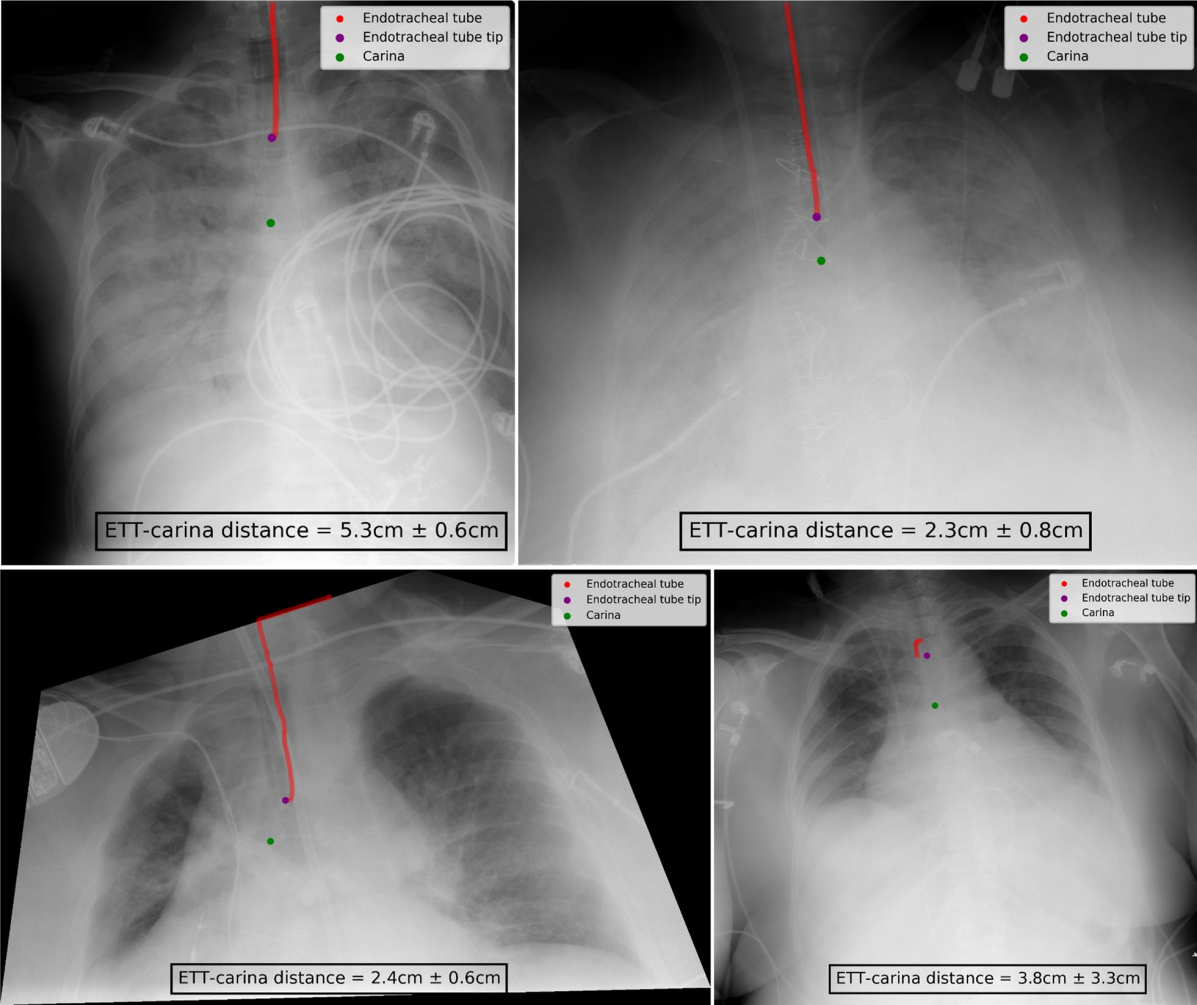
Example outputs of the CarinaNet model. Top-left and top-right chest radiograph were, respectively, classified as having *acceptable quality* and a *hardly visible carina*. The endotracheal tube tip and carina were accurately detected and the model uncertainty was inferior to 1 cm, indicating that the model result could be trusted. The segmentation was successful for both these radiographs. Bottom-left radiograph had a very unusual angle, this disrupted the segmentation step. However the endotracheal tube was entirely segmented and the endotracheal tube tip and carina were accurately detected. Bottom-right radiograph featured no endotracheal tube. The CarinaNet model properly detected the carina and output an endotracheal tube tip position but with an uncertainty of 3.3 cm indicating that the result could not be trusted. Note that the segmentation step also failed
